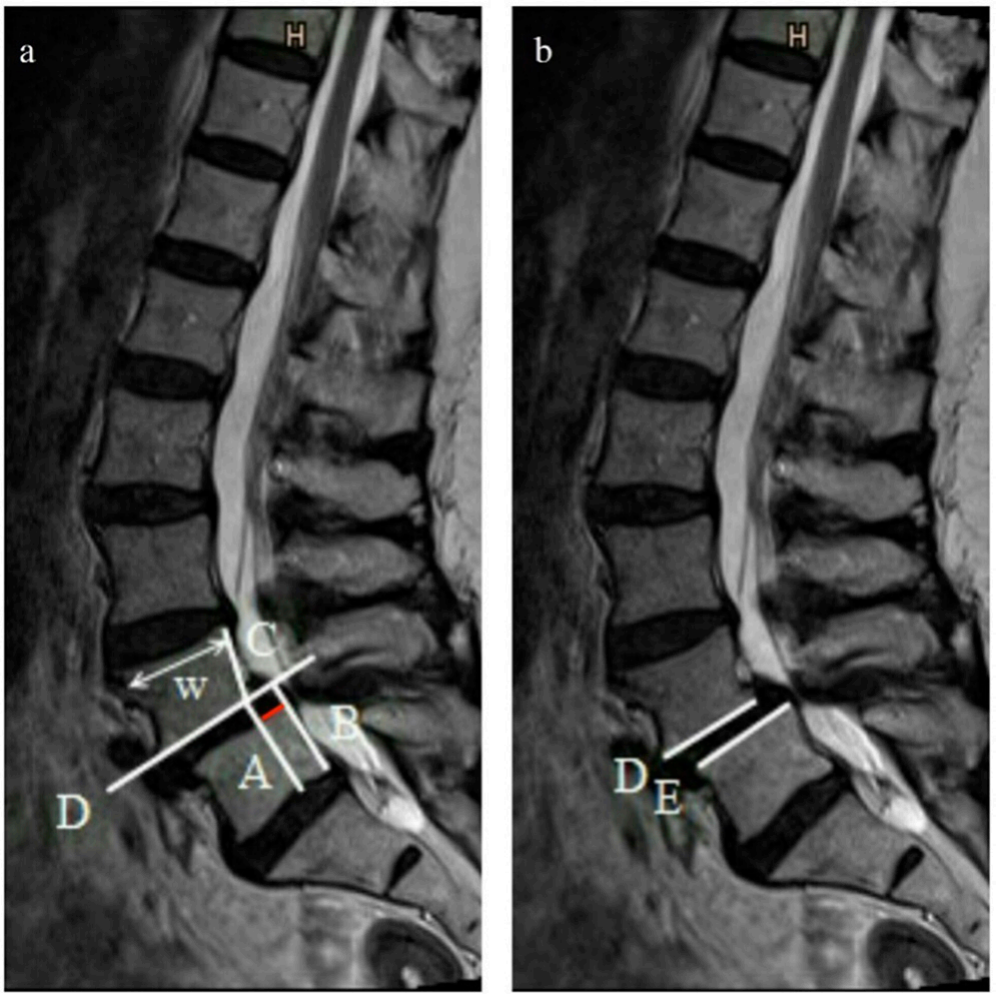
Measurement technique in a sagittal T2-weighted MRI: in figure a, the red line is the distance between line A and B and represents the sagittal translation (ST). The sagittal rotation (SR) is the angle between line D and E shown in figure b.
